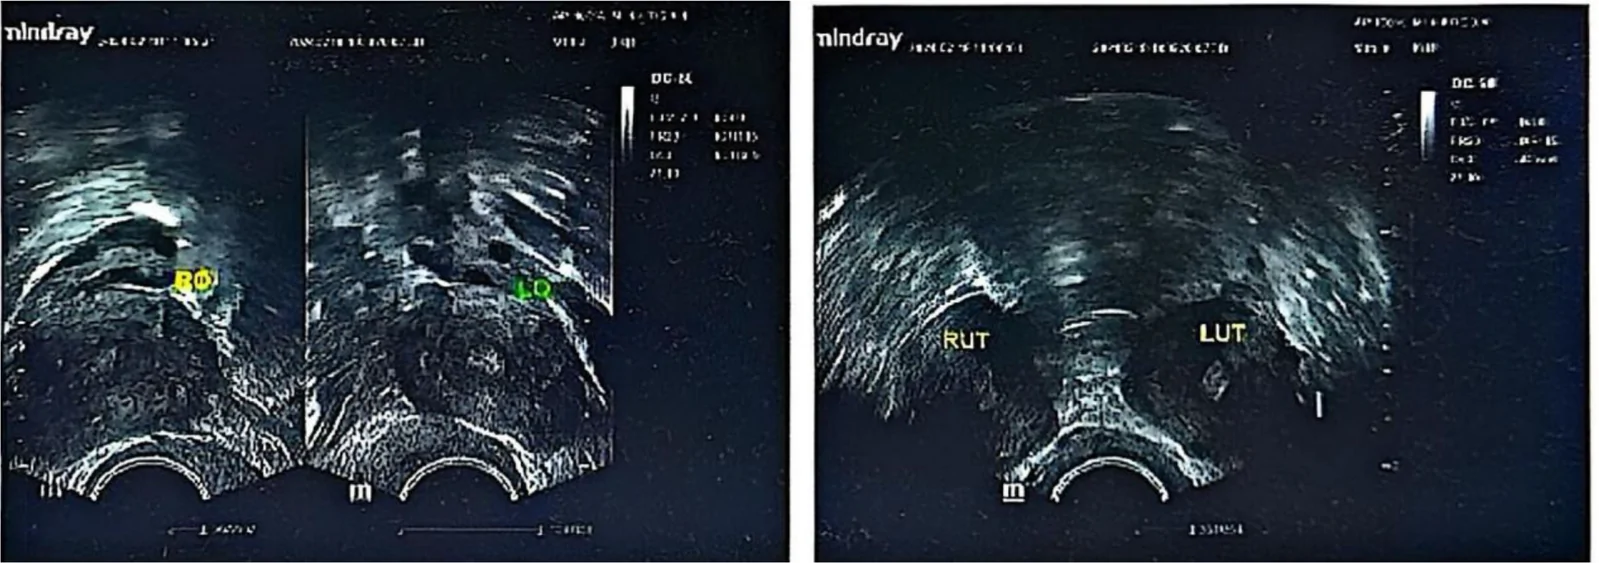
Prepregnancy ultrasound examination. LO = left ovary, LUT = left uterine tube, RO = right ovary, RUT = right uterine tube.
